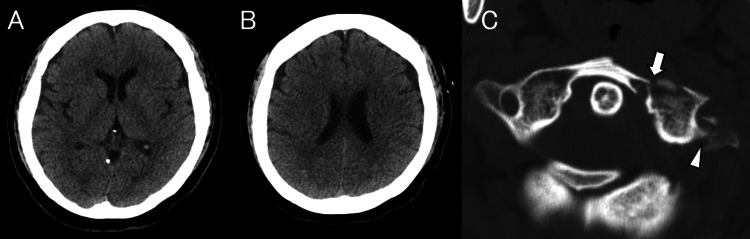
Initial head and cervical CT findings at the referring hospital. Non-contrast head CT demonstrating no skull fracture or intracranial hemorrhage (A, B). Cervical CT showing a fracture of the anterior arch of the atlas (white arrow) and a transverse process fracture extending to the left transverse foramen (arrowhead) (C).
